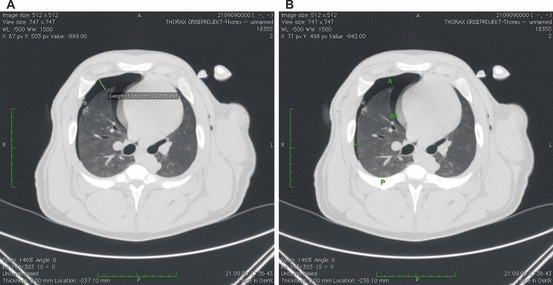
CT scan of a PTX, (A) Measurement of the LE-CW distance. (B) Intrathoracic distribution of the PTX. Air is in the anterior, medial, and lateral locations with no air posterior to the lung parenchyma. CT = computed tomography; LE-CW = lung edge–chest wall; PTX = pneumothorax.
